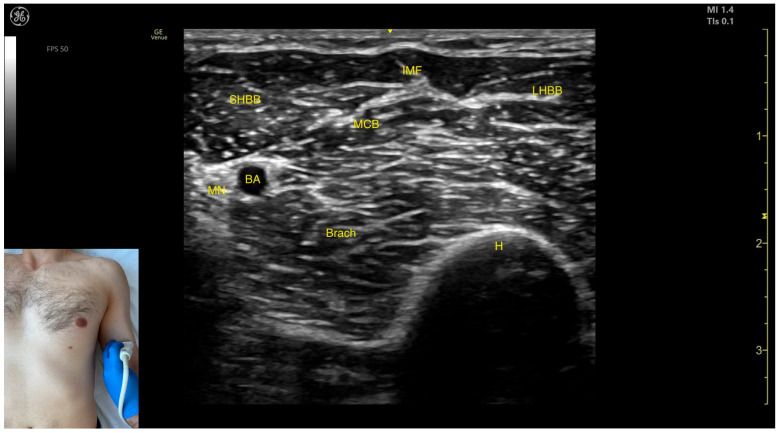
Ultrasound anatomy of the biceps brachii (BB) with key landmarks: SHBB—short head biceps brachii; LHBB—long head biceps brachii; IMF—intramuscular fascia; MCB—musculocutaneous branch; MN—median nerve; BA—brachial artery; Brach—brachialis; and H—humerus.
